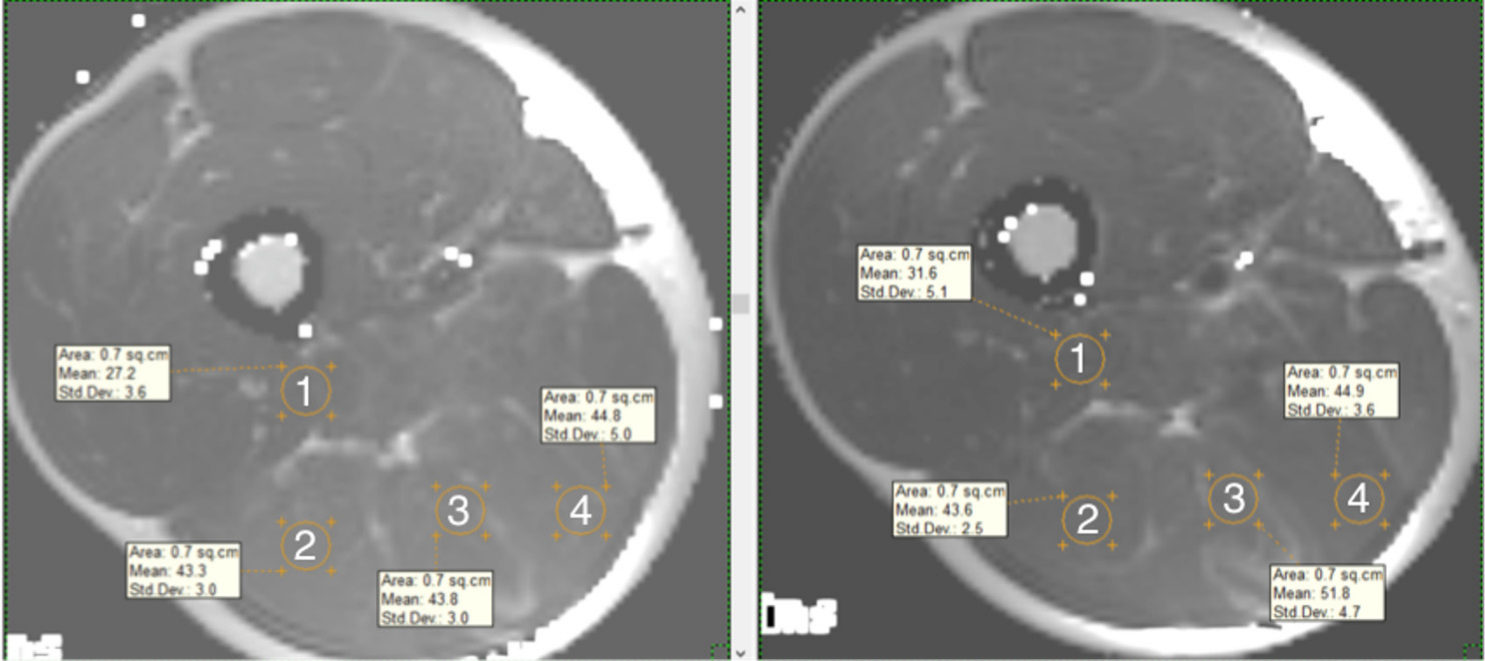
Selected MR images, obtained PRE and POST exercise, depicting regions of interest (ROIs). 1; m. biceps femoris short head, 2; m. biceps femoris long head, 3; m. semitendinosus, 4; m. semimembranosus.
